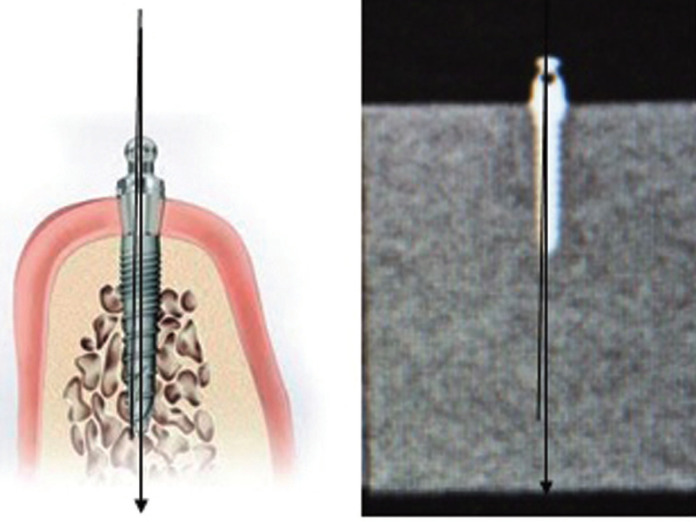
a) Pictorial representation of deflection of mini implants. b) Radiographical evaluation of mini implants.
